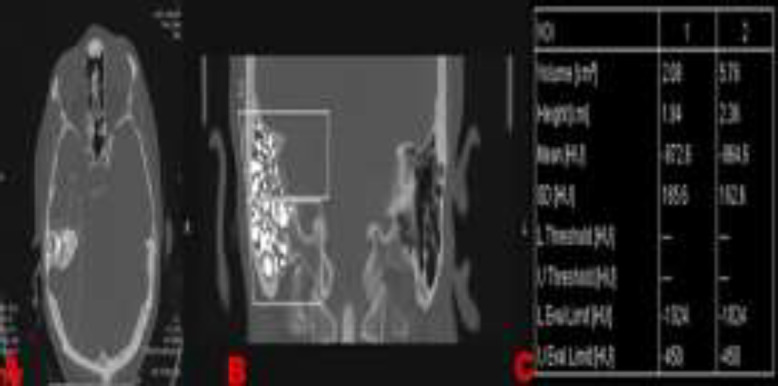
(a) The measurement of right mastoid air cell volume on the computed tomography (CT) axial slice with a gray-scale similar to air. (b) The measurement of right mastoid air cell volume on the CT coronal slice with a gray-scale level similar to air. (c) Calculation of the areas and the volumes of the extracted pneumatized parts of mastoid bone
